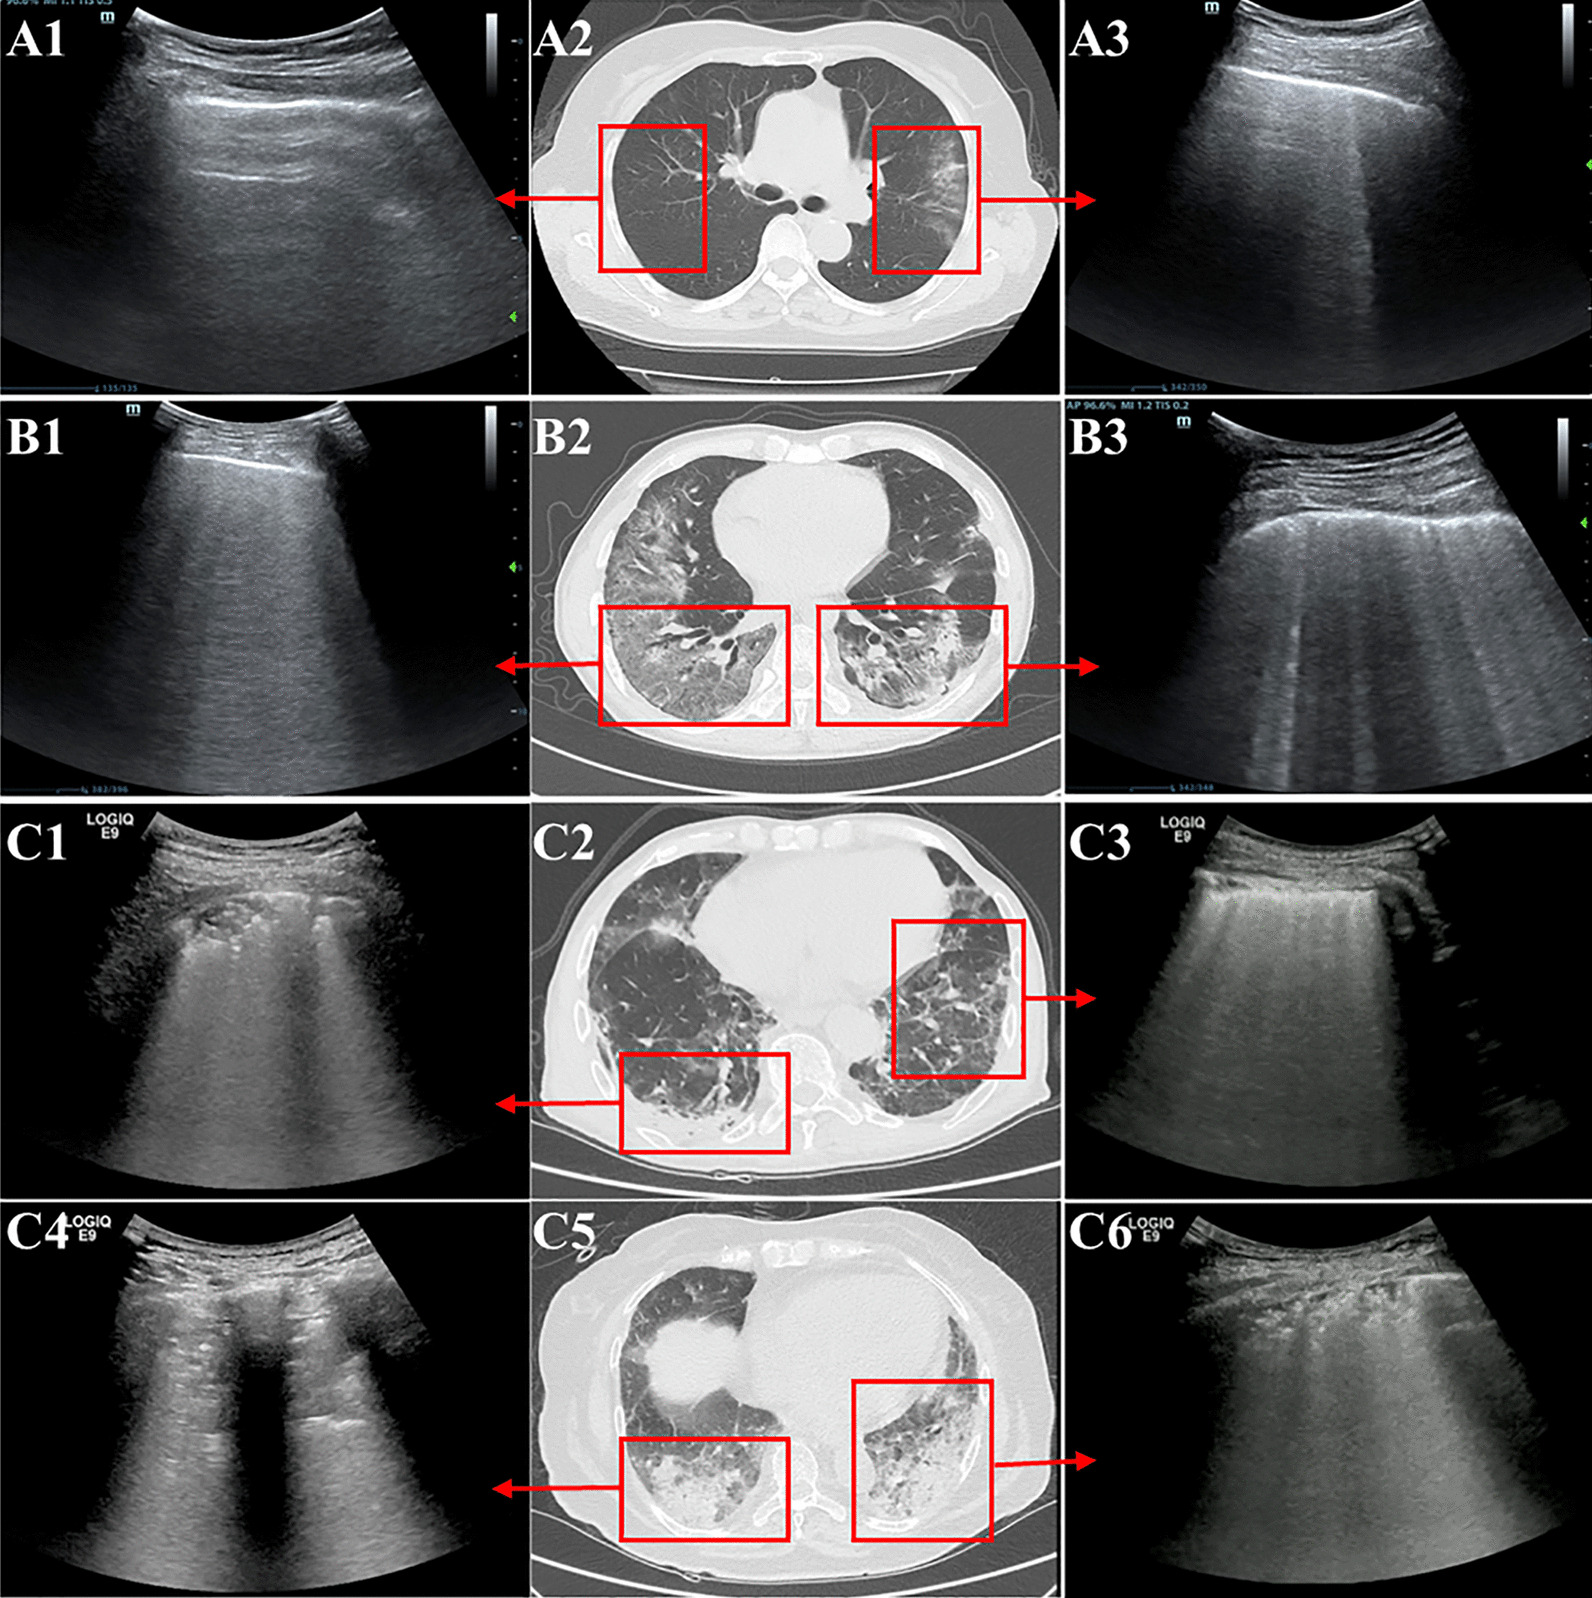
Typical lung ultrasound and corresponding lung CT images of patients with various LUS score. A: Lung images of a patient with a low LUS score; A1: normal pleural line and no B-line, score 0; A3: normal pleural line and well-spaced B-lines ≥ 3, score 1; A2: lung CT in the corresponding areas. B: Lung images of a patient with a moderate LUS score; B1: normal pleural line and multiple coalescent B-lines, score 2; B3: irregular pleural line and well-spaced B-lines ≥ 3, score 2; B2: lung CT in the corresponding areas. C: Lung images of a patient with a high LUS score; C1: irregular pleural line and lung consolidation, score 4; C3: blurred pleural line and multiple coalescent B-lines, score 4; C4: blurred pleural line and lung consolidation, score 5; C6: irregular pleural line and lung consolidation, score 4; C2, C5: lung CT in the corresponding areas. (Note: Lung images of all 12 regions of each patient are not shown in the figure. The total LUS score should be calculated with a total score of 12 regions.)
